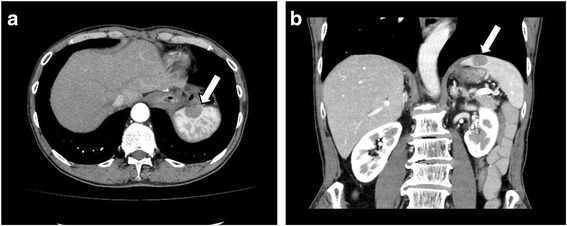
The CT scan reveals a low-density lesion that is 17 mm in diameter and at the upper pole of the spleen (*arrow*). a: The horizontal section. b: The coronal section
